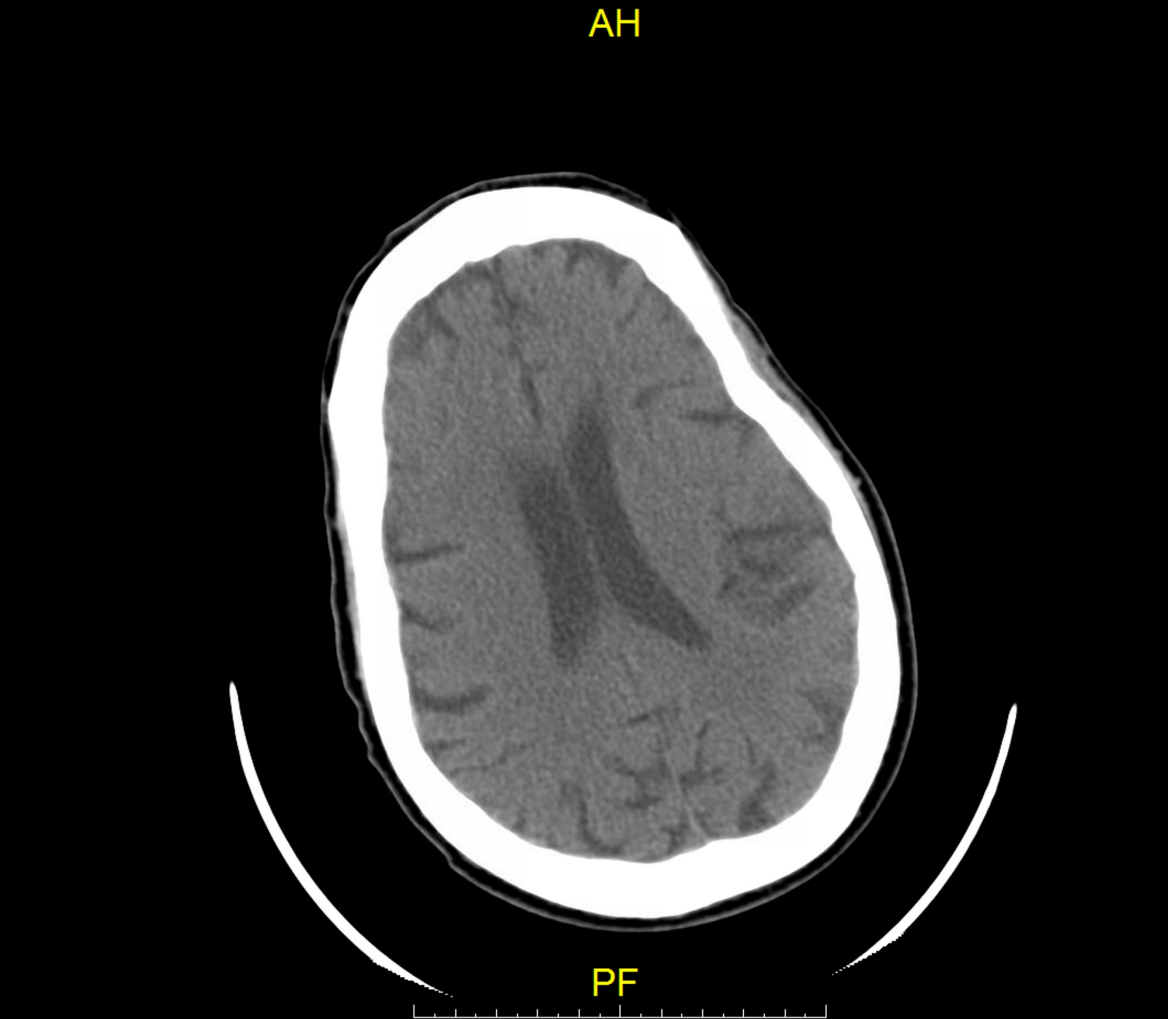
Initial CT head without contrast on arrival negative for acute infarct or hemorrhage
